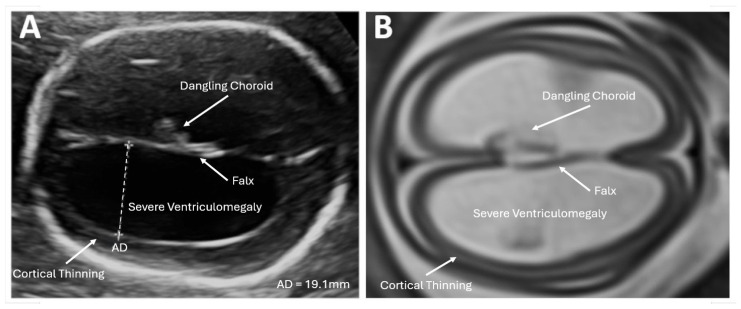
Imaging in the transventricular plane of ISVM at 22.3 weeks of pregnancy: (A) transabdominal ultrasound: the atrial width of the distal ventricle is increased at AD = 19.1 mm, and (B) MRI of T2-dependent sequence SSFSE.
